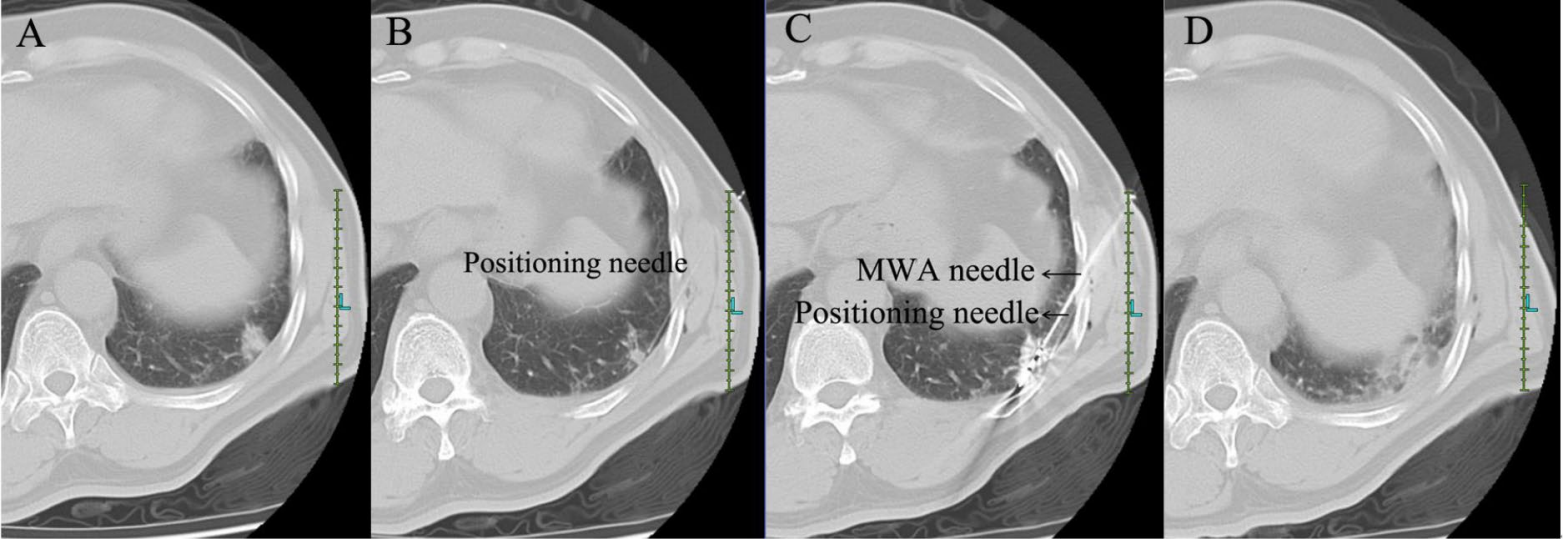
A clinical MWA puncture case by applying a fine needle-mediated breathing control technique for a 59-year old male who had esophageal carcinoma history. (A) Routine CT scan showed the feature of the lesion; 1.7 cm × 1.6 cm × 2.0 cm in size and located at posterior basal segment of lower lobe of the left lung. (B) A 23G fine needle was inserted into the lung as breathing indicator, showing the needle paralleled to the CT cross section and the whole length of needle visible in the image. (C) With the reference of the fine needle, a MWA electrode was inserted to the distal margin of the lesion. (D) Post-ablation CT scan observed no obvious complications.
